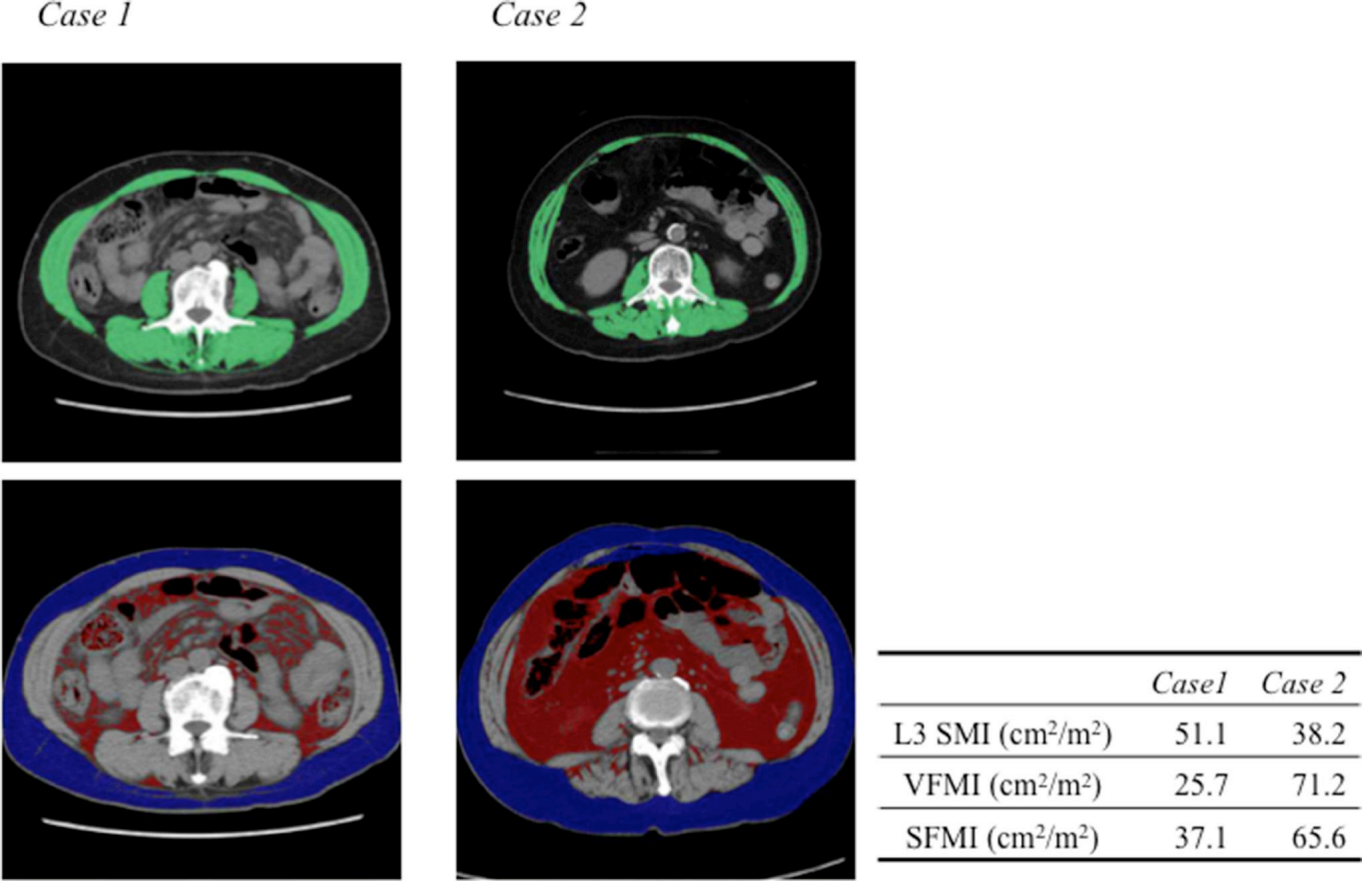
Computed tomographic images used for the assessment of L3 skeletal muscle index (SMI), visceral fat mass index (VFMI), and subcutaneous fat mass index (SFMI) Two cases of female HCC patients are shown. The body mass indices of the two patients were identical (25 kg/m^2^). Case 1 is a typical patient with higher SMI and lower VFMI and SFMI. Case 2 is a typical patient with lower L3 SMI and higher VFMI and SFMI. The green, red, and blue shading indicate skeletal muscle, visceral fat, and subcutaneous fat, respectively.
